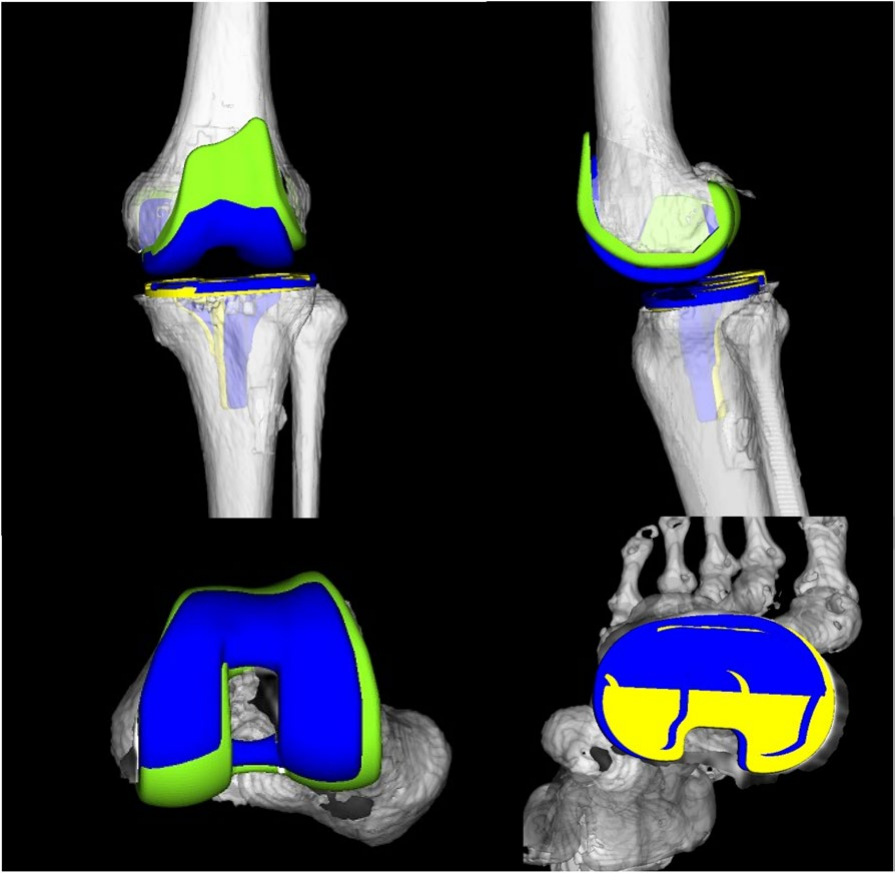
The preoperative three-dimensional computed tomography image (3DCT) plan and a postoperative 3DCT image are shown. 3D computer-aided design data of femoral and tibial components were fit to the 3DCT image using six parameters, specifically the coronal, sagittal, and axial alignment of the femoral and tibial prostheses. The blue components indicate the preoperative plan image; the green and yellow components indicate the postoperative component
